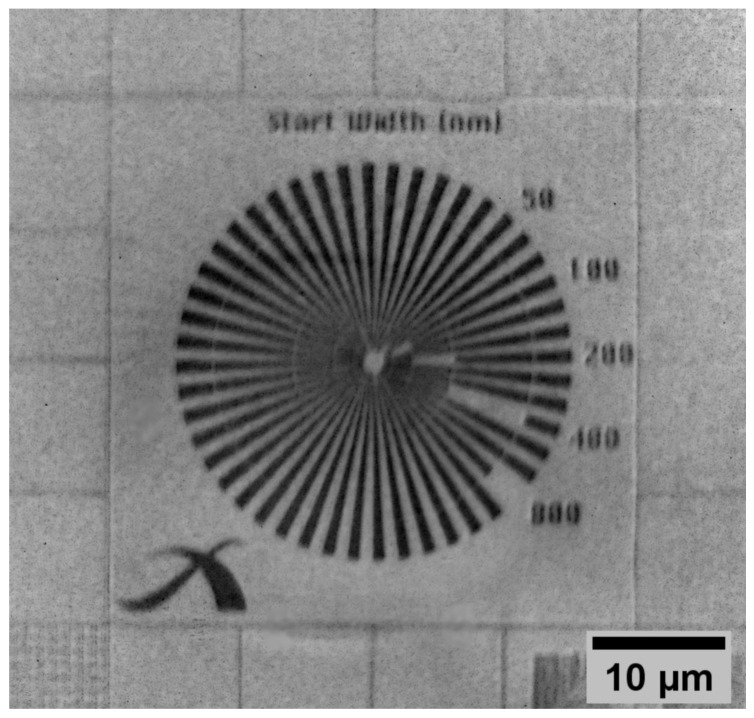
X-ray image of a Siemens star test structure (photon energy 9.2 keV, average of 10 images, acquisition time for each image 120 s, background and flatfield corrected, no denoising, 1024 px × 1024 px). The notches in the imaged Siemens star design start with a width of 50 nm (in the center) and are increased clockwise gradually to 100 nm, 200 nm, 400 nm and finally 800 nm.
